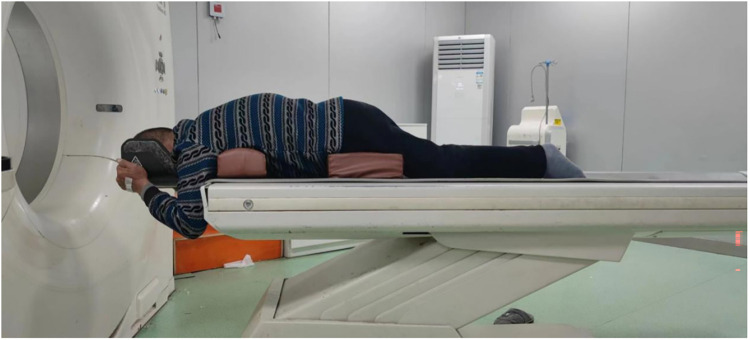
Patient positioning for full-length spine CT scout view.
